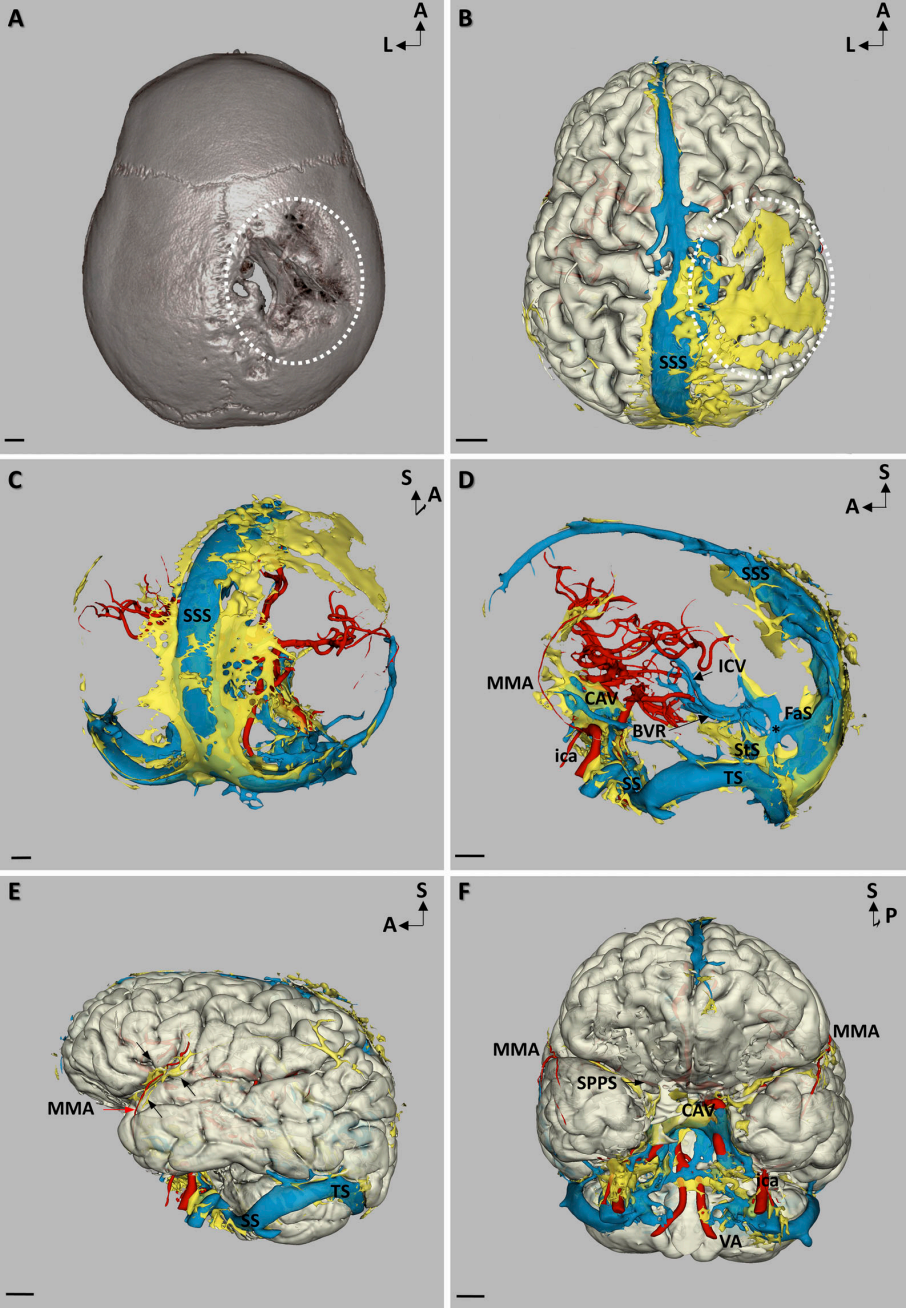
Altered MLV imaging overlaps with skull bone erosion in a patient with GSD. (A) 3D reconstruction of a computed tomography scan in superior view showing the large erosion of the right parietal bone (white circle). (B–F) Meningeal vascular 3D mapping by MRI. (B) Superior view showing a large area of gadobutrol slow-flow (yellow) in the region of the vanished bone (white circle) and extending to the perisinusal area of the SSS. (C) Posterior view showing the extension of the perisinusal gadobutrol enhancement (yellow) all along the SSS. (D) Lateral view showing that MLV hypertrophy involved MLVs of the SSS, StS, LS, SS, and CAV. This MLV hypertrophy was associated with a malformation of the StS that was replaced by a large venous plexus (asterisk) encompassing the StS and an abnormally persistent embryonic falcine sinus (FaS). (E) Lateral view reconstruction highlighting the close relationships between the MMA (red arrow) and its related MLVs (black arrows). (F) Anterior view allowing the visualization of the CAV, ica, and vertebral arteries (VA). MLV hypertrophy allowed to visualize the continuity of MMA-related MLVs toward the skull base. Note that, instead of lining the MMA toward the spinosum foramen, MLVs followed the middle meningeal vein toward the sphenoparietal sinus (SPPS) toward the CAV. Scale bar: 1 cm (A–F).
